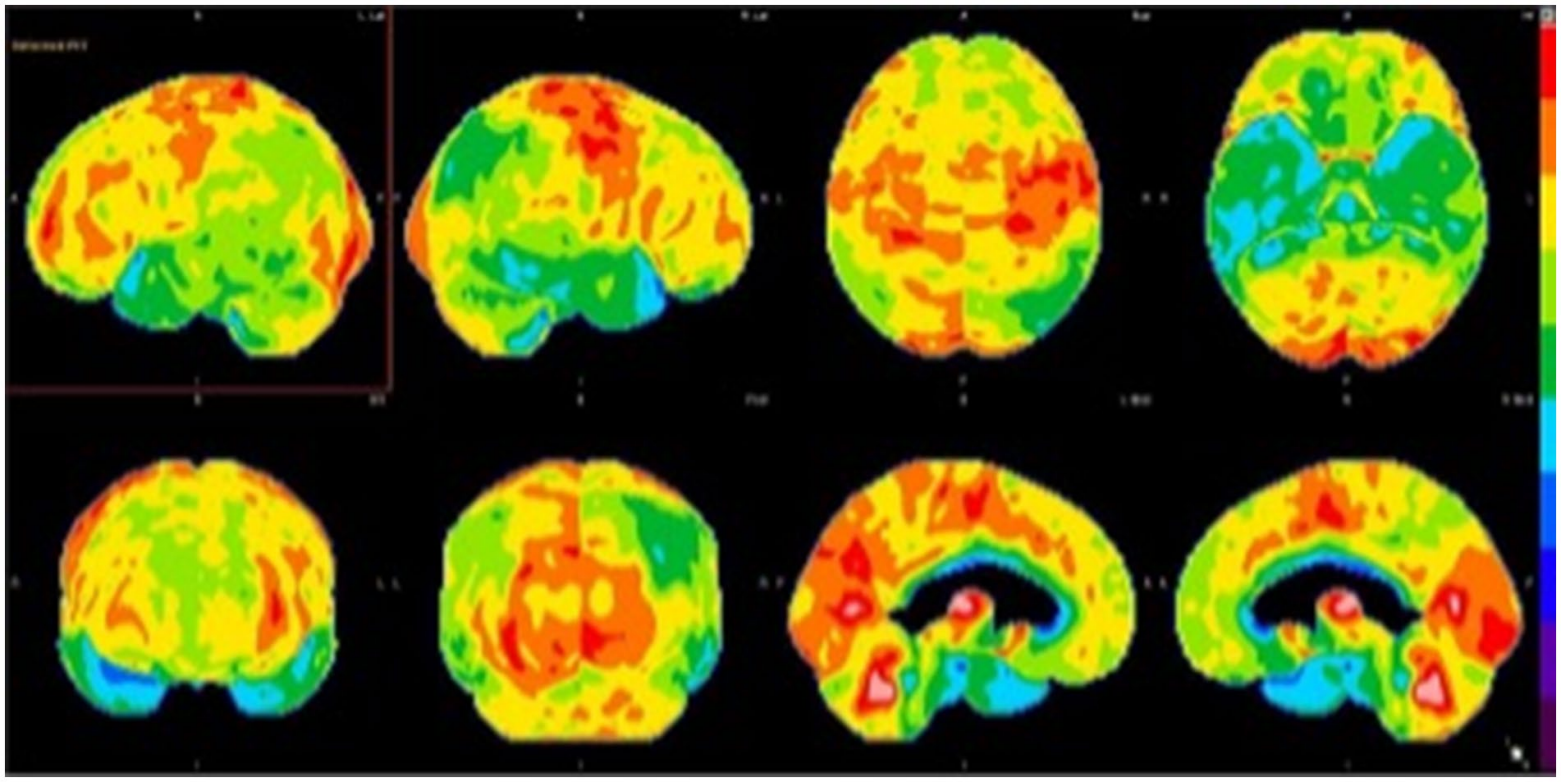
Brain FDG-PET imaging: 3D stereotactic surface projection maps demonstrate moderate hypometabolism in the parietotemporal regions (right greater than left), which extends into the frontal lobes. There is accompanying mild hypometabolism in the precuneus and posterior cingulate gyri, especially on the right side, regions typically involved early in the setting of Alzheimer’s disease.
